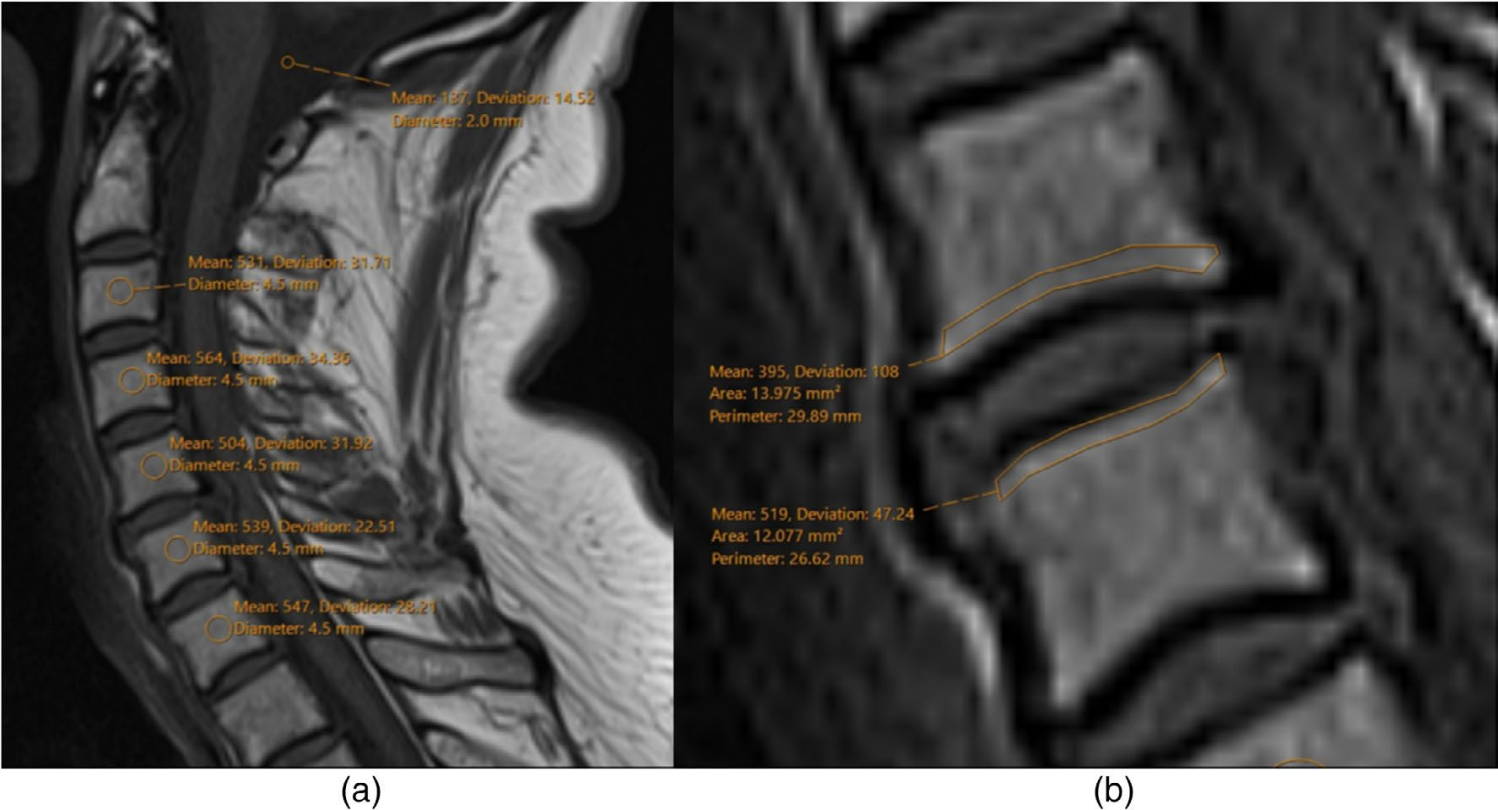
VBQ measured across C3–7 with CSF standardisation at the Cisterna Magna (a) and EBQ score measurement at operated levels (b) from preoperative mid‐sagittal MRI.
